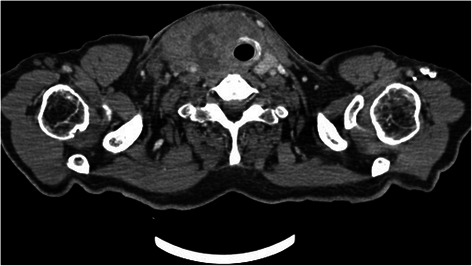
CT showing a large heterogeneous mass of the thyroid gland
